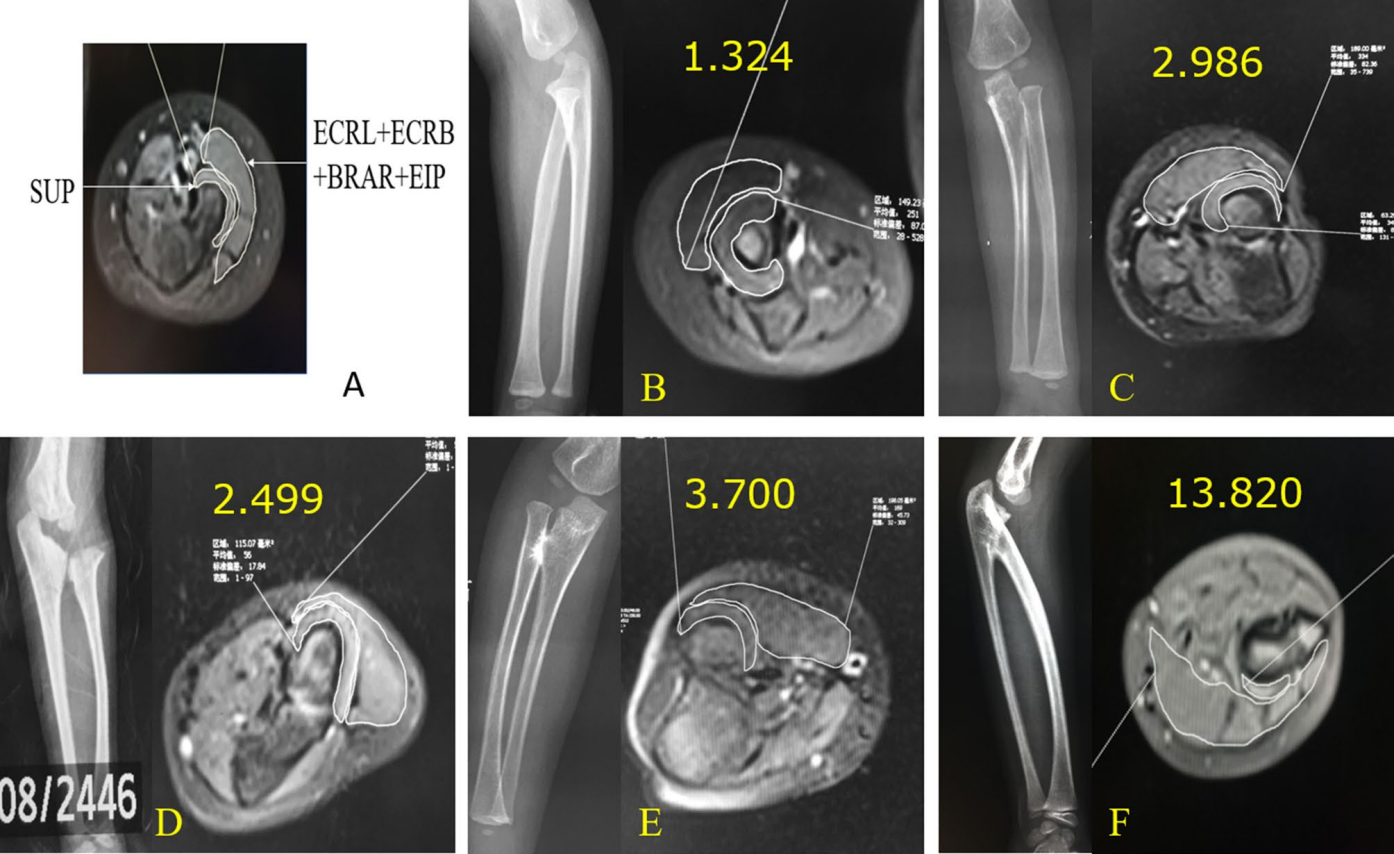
(A) The muscle selection of (ECRL + ECRB + BRAR + EIP) and supinator. (B) Radiography and MR image of the normal forearm with a supinator ratio of 1.324. (C) X-ray, MR image and supinator ratio of MRI & X-ray type I forearm, also classified as Cleary & Omer type I: Fibrous pseudo-synostosis with any shape of the radial head. (D) Radiography, MR image and supinator ratio of MRI & X-ray type I forearm, also classified as Cleary & Omer type IV. (E) Radiography, MR image and supinator ratio of MRI & X-ray type II: radiographic osseous synostosis with radial head dislocated. (F) Radiography, MR image and supinator ratio of MRI & X-ray type III, Tachdjian type I: radiographic radial head unobservable and osseous synostosis with the ulna.
